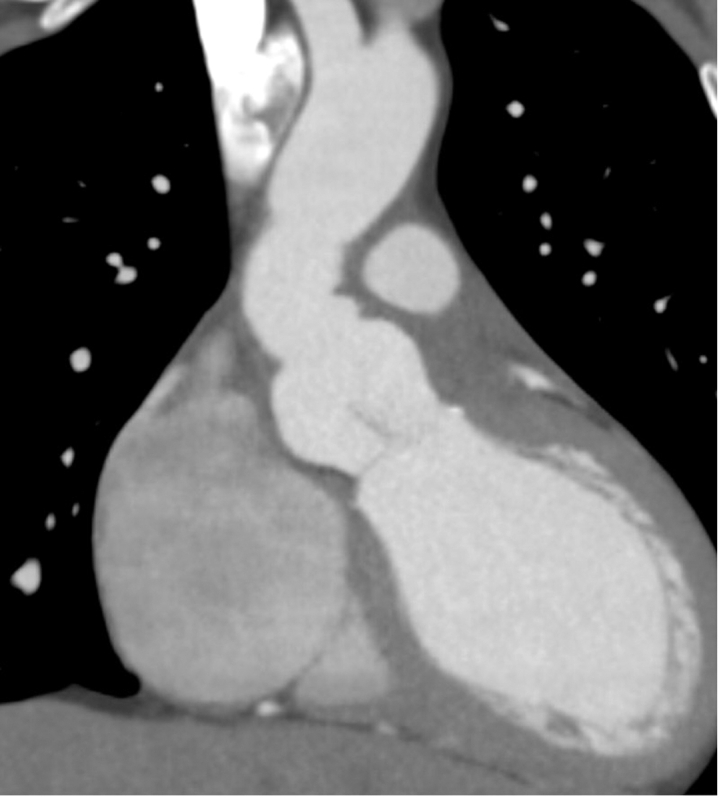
Postoperative computed tomography angiography of autograft 1 year after the Ross with the Seattle shawl technique. The autograft measures 3.7 cm in diameter.
